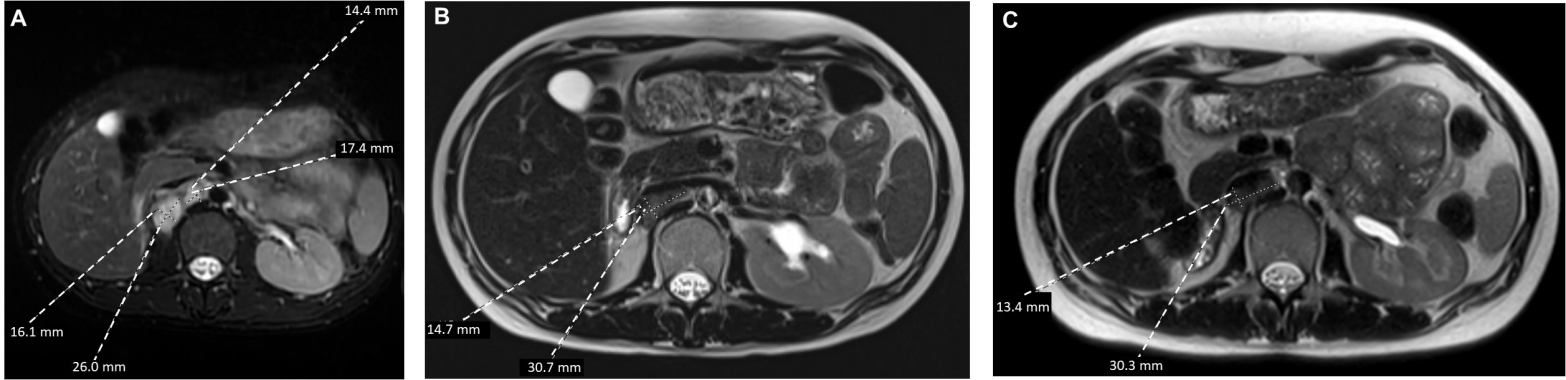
Axial T2-weighted MRI view of the retroperitoneal metastasis (A) at baseline, (B) following four cycles of doxorubicin plus cyclophosphamide, and (C) following six infusions of weekly paclitaxel.
